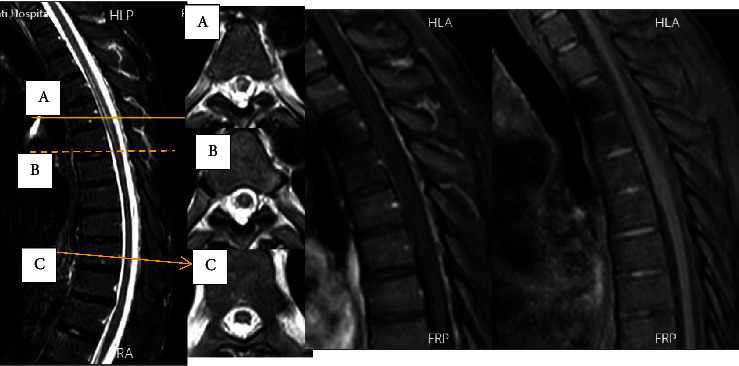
Thoracic Cord Imaging. The leftmost image represents the midsagittal section of the thoracic cord. The subsequent series of pictures display axial views of three distinct segments of the spinal cord (labeled as A, B, and C) corresponding to the midsagittal section. The third image depicts the T1-weighted sagittal section without contrast injection. The image on the right shows the T1-weighted sagittal section after gadolinium injection.
